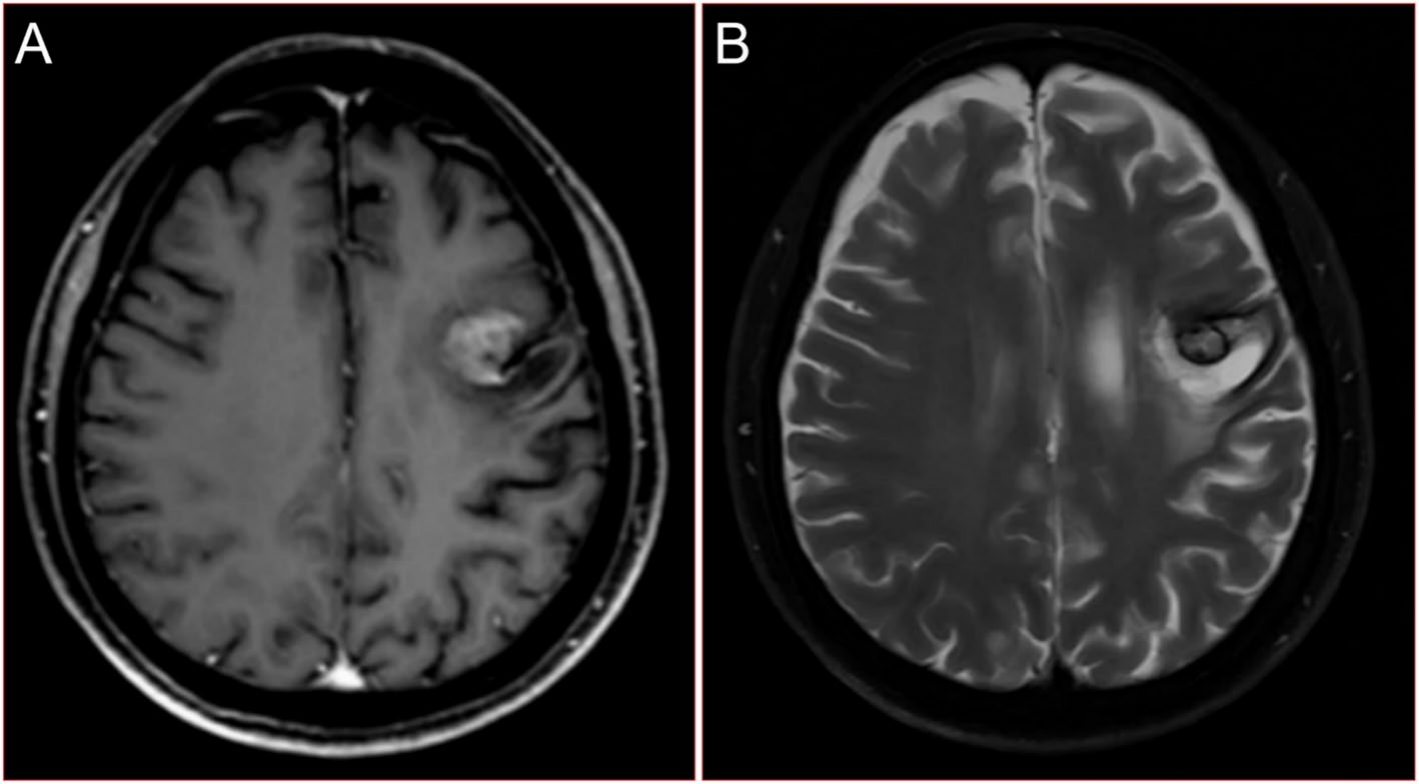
Reexamination magnetic resonance images of the head after chemotherapy of reexamination of the patient. (A) Contrast enhanced T1WI. (B) T2WI.
